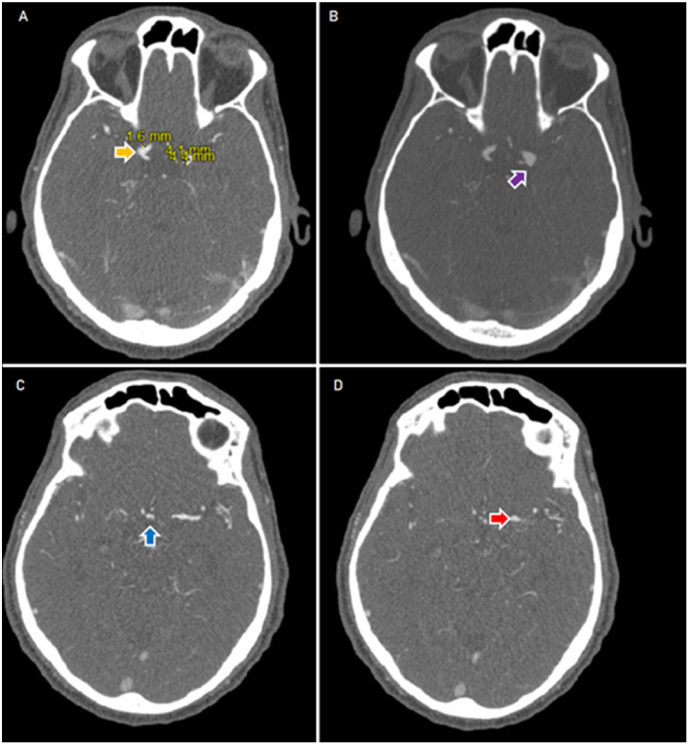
Computed Tomography angiogram demonstrating intracranial aneurysms in various anatomical locations. (A) Right terminal internal carotid artery (yellow), broad-based, measuring 1–2 mm projecting posteriorly (B) 4 × 4 mm broad-based left posterior communicating artery aneurysm (purple) projecting posteriorly (C) A 3 mm broad-based right anterior communicating artery aneurysm (blue) (D) A 3 mm broad-based aneurysm projecting superiorly from the left carotid bifurcations (red). (For interpretation of the references to colour in this figure legend, the reader is referred to the Web version of this article.)
